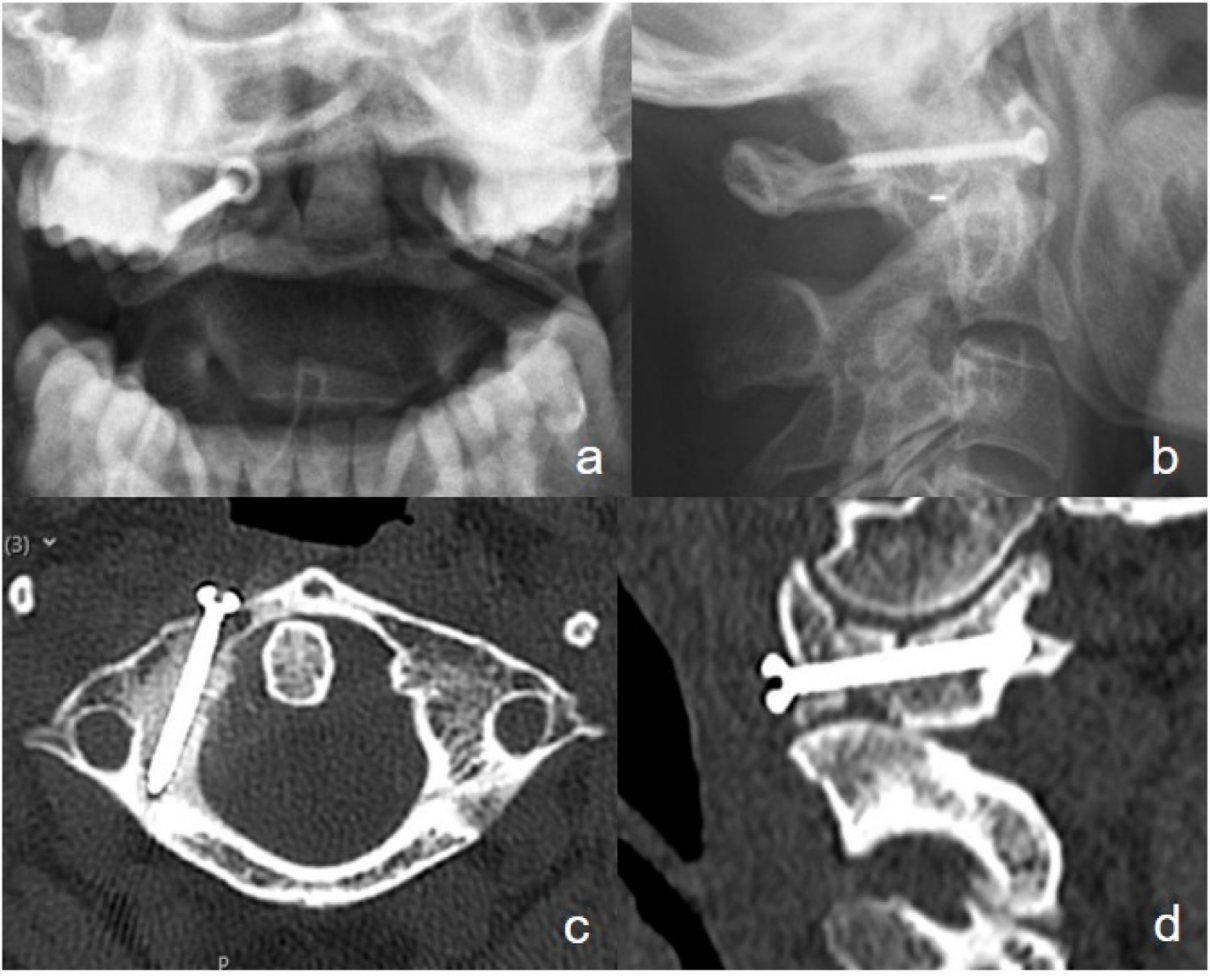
Follow-up x-ray (a/b) and CT-scan (c/d) after six weeks with correct screw position and no loss of reduction.
